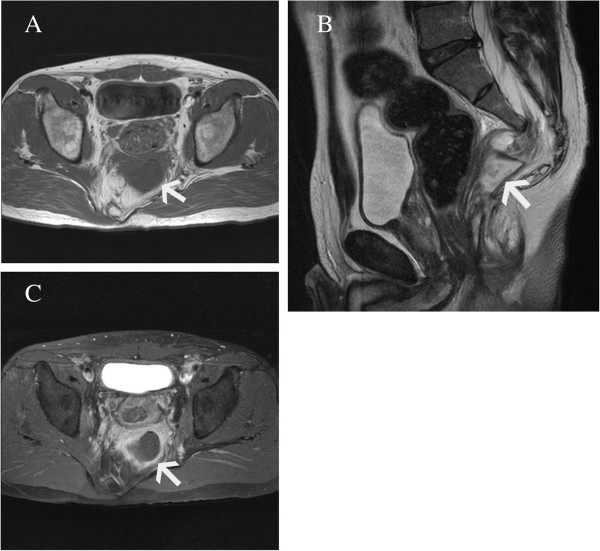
Magnetic resonance imaging findings. (A) Axial T1-weighted image showing a low density of the presacral mass (white arrow). (B) A tethered cord could not be revealed on a sagittal T2-weighted image. (C) The mass was not enhanced on contrast-enhanced magnetic resonance imaging. The cystic wall was thick, surrounding a fuzzy tissue.
